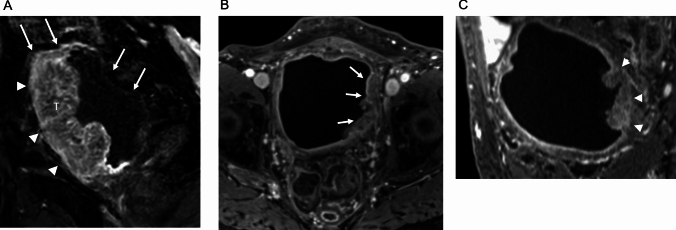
Cases of muscle-invasive bladder cancer. A Dynamic contrast-enhanced MRI (DCE-MRI) acquired using Cartesian technique at 90 s post-contrast injection. This DCE-MRI shows significant intestinal motion artifacts on both the superior and posterior walls of the bladder (arrows). A widespread tumor (labeled as T) is visible on the anterior wall of the bladder. The tumor projects beyond the bladder wall, suggesting infiltration into the adipose tissue (arrowheads). The pathological findings from transurethral resection of the bladder tumor (TURBT) indicated a stage of T2 or higher. B DCE-MRI acquired using GRASP technique, axial section at 90 s post-contrast injection. The tumor is located on the left wall of the bladder (arrows) and is broad-based. C Reconstructed cross-sectional image at the intersection of tumor and muscle layer. This image clearly shows disruption of the inner layer enhancement at the base of the tumor (arrowheads). Pathological examination from TURBT confirmed a stage of T2 or higher
